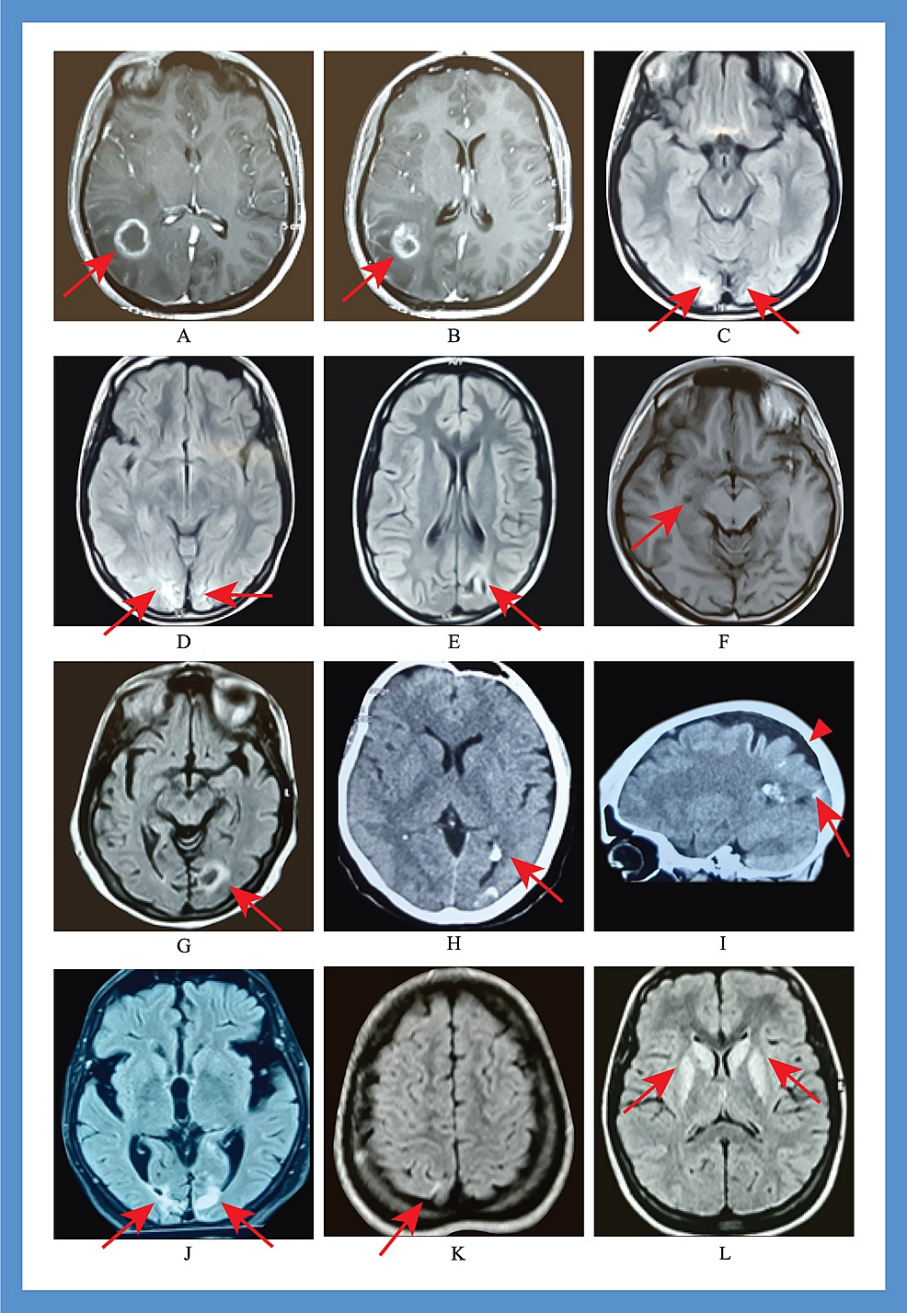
Brain imaging of the different patients with occipital epilepsy (A, B) MRI of the brain with contrast showing multiple rings enhancing lesions in the right parieto-occipital region (case 5; red arrow); (C, D) MRI of the brain showing sclerosis in both occipital poles marked on the right side (case 8; red arrow; (E) MRI of the brain showing focal cortical dysplasia in the left occipital region (case 1; red arrow); (F) MRI of the brain showing arachnoid cyst in the right hippocampal region (case 2; red arrow); (G) MRI of the brain with encephalomalacia and marginal sclerosis in the left occipital region (case 7; red arrow); (H, I) CT scan of the brain showing gyral calcification in the left occipital region (case 10; red arrow) and associated cortical atrophy (arrowhead); (J) MRI of the brain showing sclerosis in the occipital region (case 8; red arrow); (K, L) MRI of the brain showing FLAIR hyperintensities in the caudate nucleus, putamen, and left occipital region (J) (case-9; red arrow).
